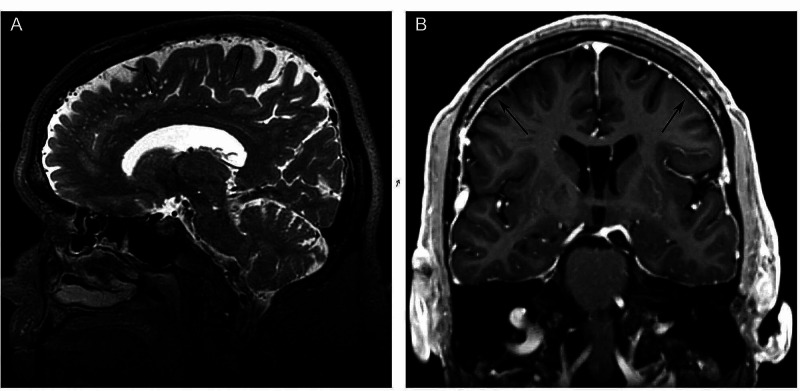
Preoperative MRI. Sagittal T2 (A) and coronal (B) T1 MRI brain with gadolinium contrast demonstrating preoperative mild, diffuse, smooth pachymeningeal enhancement without brain sag.
